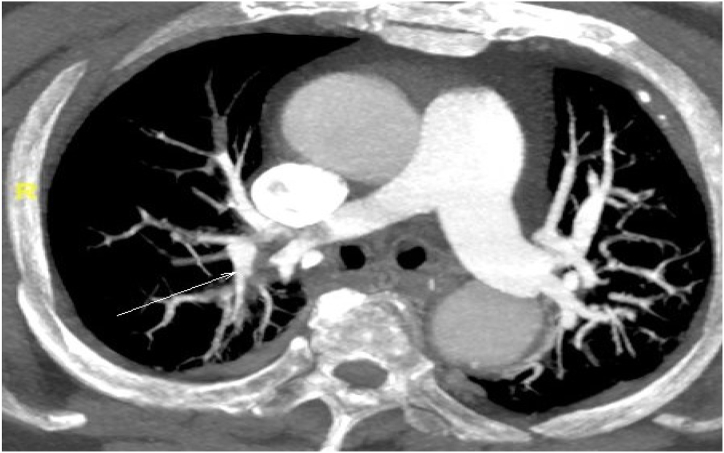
Computed tomographic pulmonary angiography: bilateral main pulmonary artery embolism.
